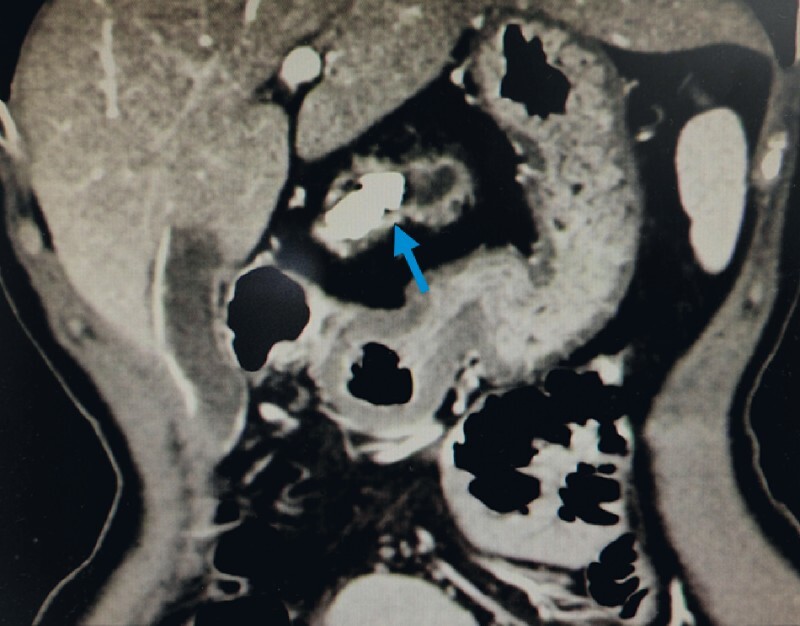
A radiopaque stone within the dilated pancreatic duct seen on computed tomography.
